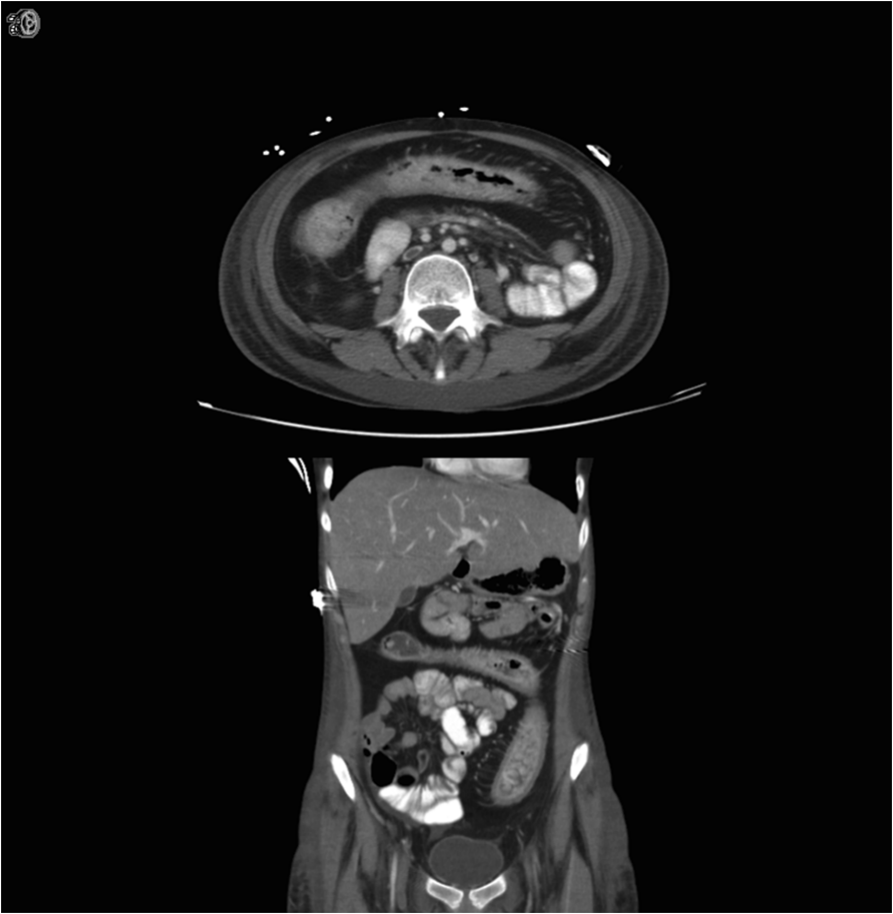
Computed tomography scan demonstrating diffuse colonic thickening.
